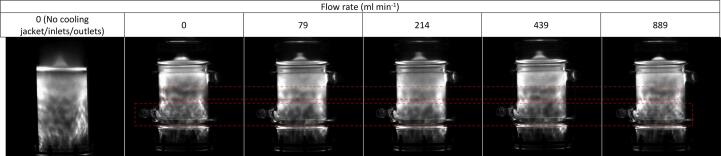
Representative sonochemiluminescence images for sonolysis of 420 ml of Milli-Q water using 410 kHz ultrasound at 80 W applied power, at liquid flow rates of 0, 0, 79, 214, 439, and 889 ml min^−1^). The image most left shows a reactor without flow, inlets, outlets, or cooling jacket, which enables the standing wave under no flow to be seen more clearly. The upper red dashed box shows the blending of a dark band with light regions into a grey band at intermediate flow rates. Similarly, the lower red dashed box shows several light and dark regions agglomerating into a few larger light and dark regions at intermediate flow rates.
